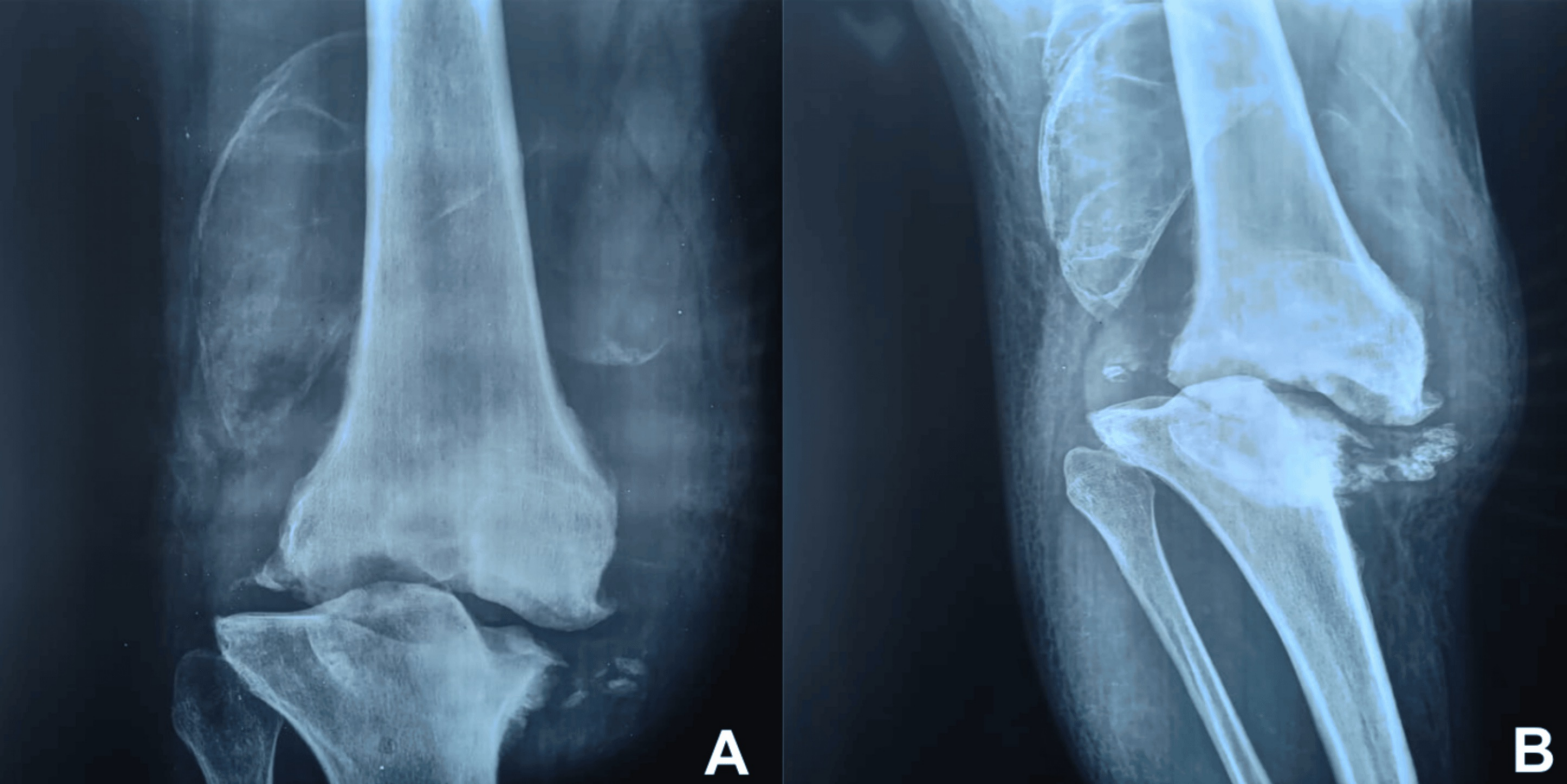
Radiographic findings of the right knee: (A) anteroposterior and (B) lateral radiographs Anteroposterior and lateral radiographs of the right knee demonstrating severe destruction of the medial femorotibial compartment, with osteolysis of the medial tibial plateau and associated osteophytic bone formations.
